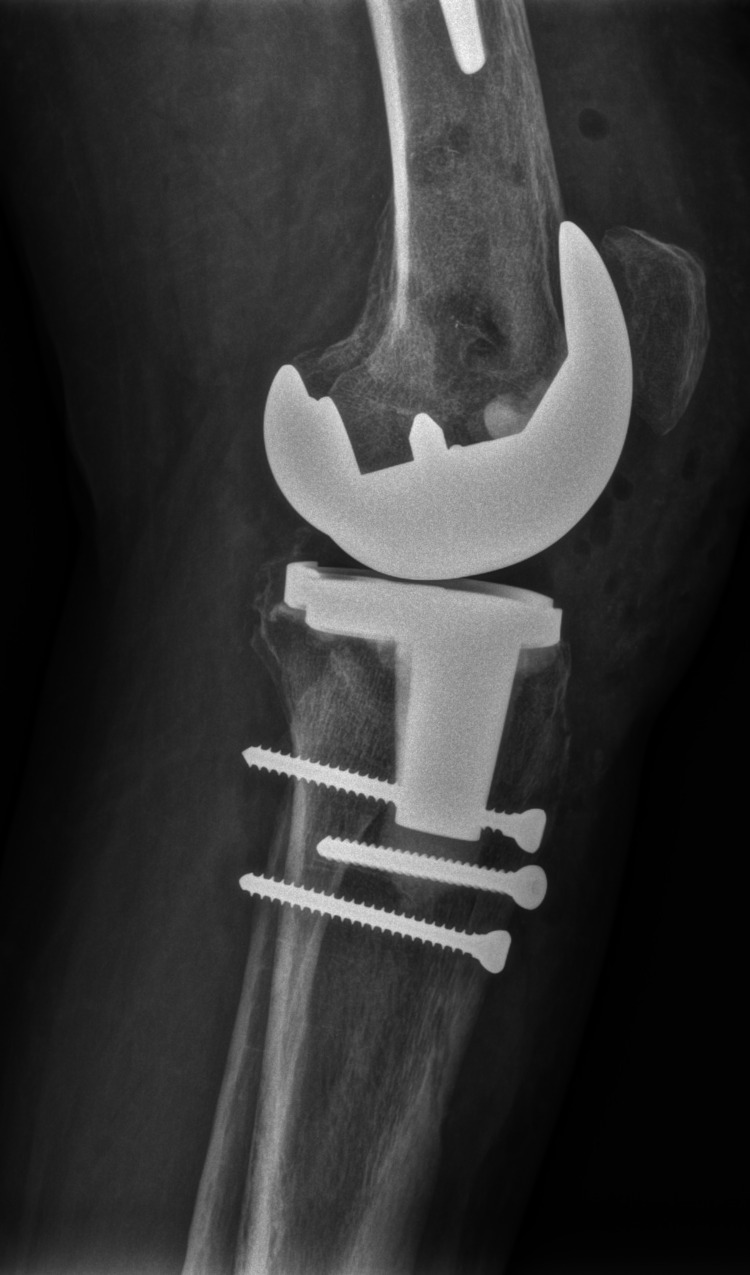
Lateral X-ray of TKA and tibial tuberosity fixation TKA: total knee arthroplasty
